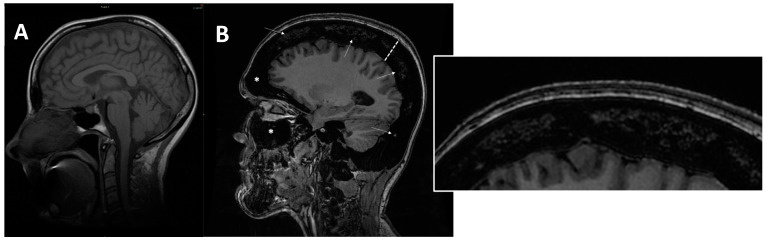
(A) axial MRI of a healthy young female (shown as comparison). (B) sagittal MRI (proton density sequence) in ARO-type osteopetrosis patient suffering from anaemia (Hb = 7g/dL) shows markedly reduced bone marrow space (arrows and enlargement on the right); diffuse calvarias bone thickening (dotted line), sclerosis (signal hypointensity), and obliteration of maxillary and frontal paranasal sinus (asterisks) can be noted.
